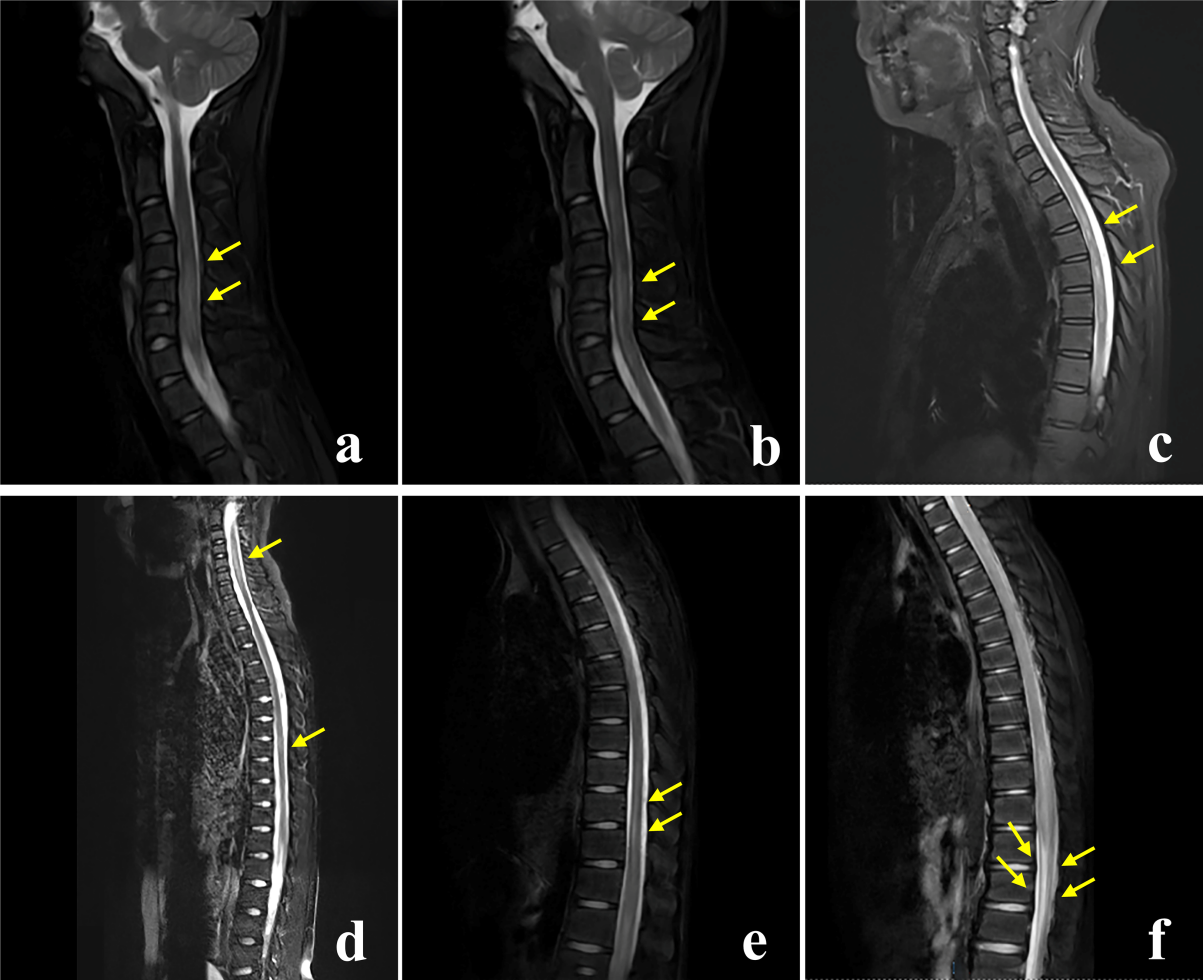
Spinal cord MRI characteristics of patients with Anti-GFAP antibodies. The MRI T2-weighted fat suppression sequence of the spinal cord in patients with anti-GFAP antibodies shows that the morphology of spinal cord lesions could be long-segment patchy lesions (A, B), multiple short-segment lesions (C), and long-segment linear lesions (D, E). Abnormal signal of conus medullaris in 1 patient (F).
